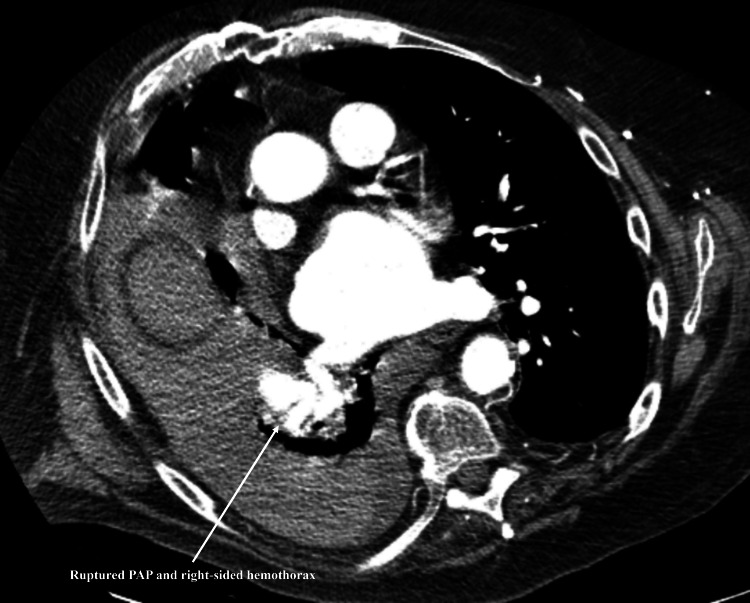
CTA chest showing right lower lobe pulmonary artery pseudoaneurysm and hemothorax PAP, pulmonary artery pseudoaneurysm
